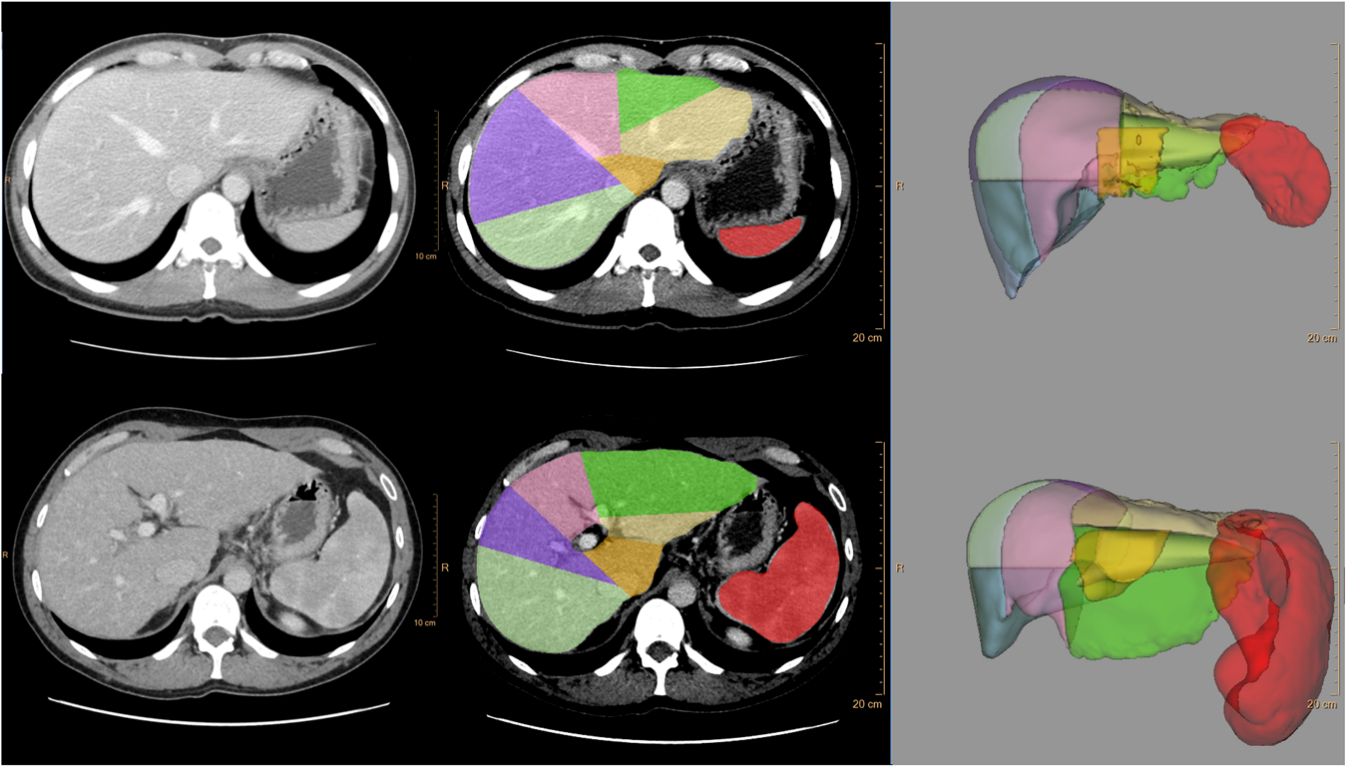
Contrast-enhanced CT with liver and spleen segmentation and 3D visualisation in a healthy subject (top row) and cirrhotic subject (bottom row)
